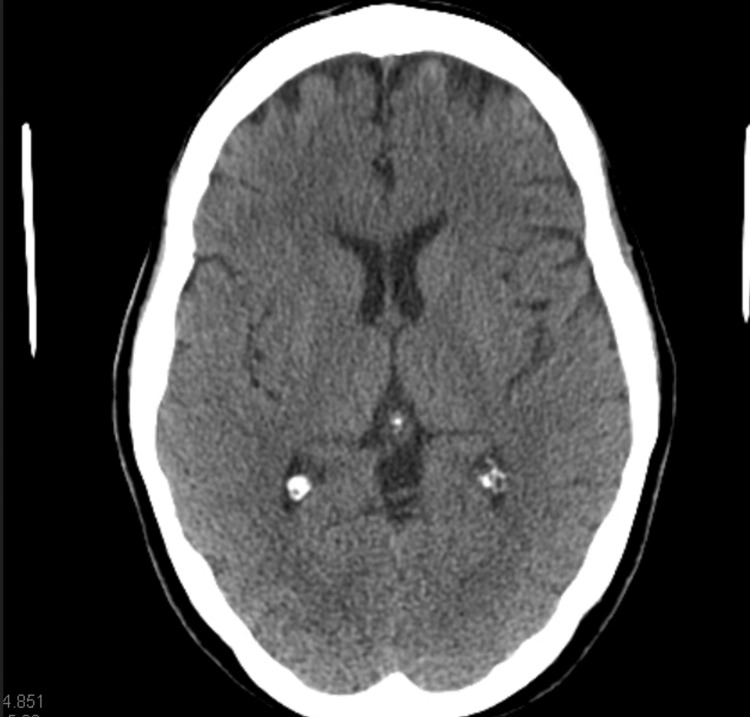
CT of the head without contrast showing no acute intracranial abnormality.
